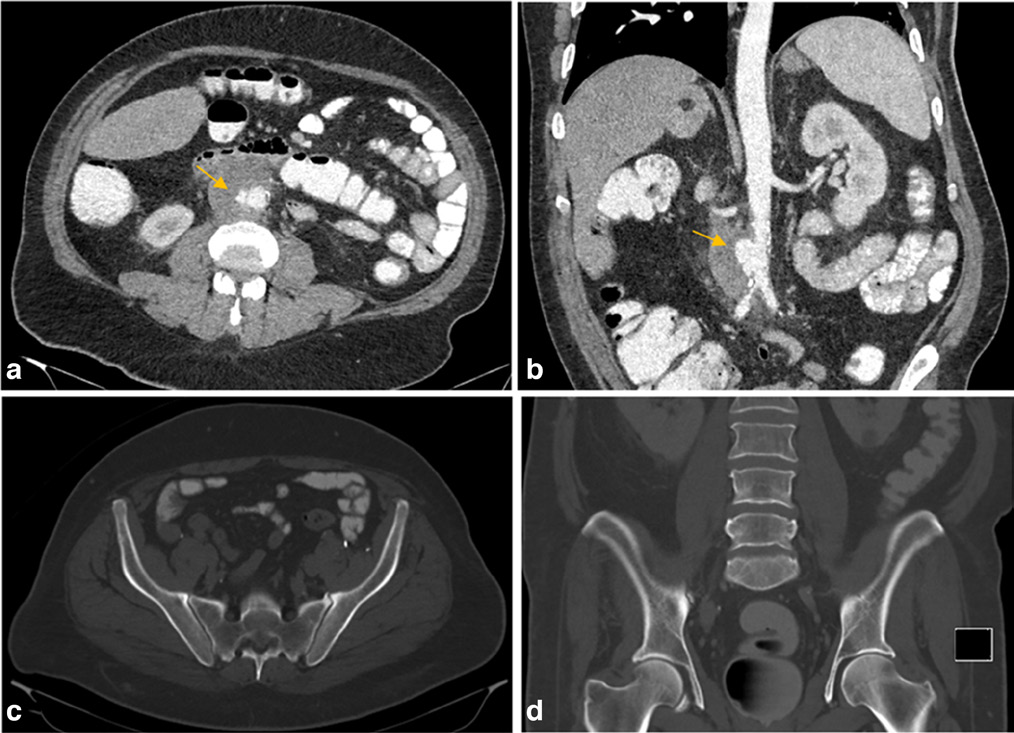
Initial CT with intravenous and oral contrast axial (a) and coronal (b) sections of the abdomen showing infrarenal aortic aneurysm with periaortic fat stranding (arrows). Axial (c) and coronal (d) bone window images show no intraosseous gas in the pelvic bones at the time of initial presentation.
